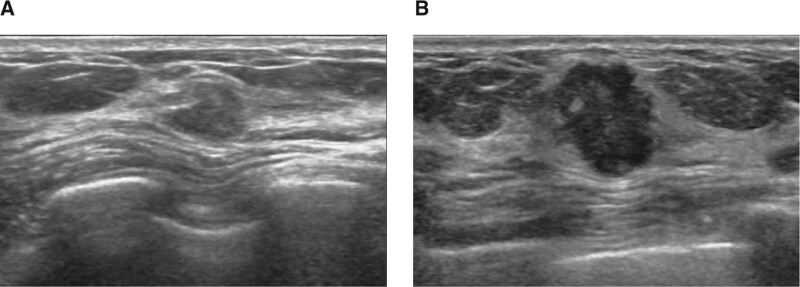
Diagnostic US for mammographic abnormality of a 68-yr-old woman. (A) Prior US shows 0.7 cm sized hypoechoic mass with an irregular shape and microlobulated margin. This mass was initially assessed as BI-RADS category 3 but reassessed as BI-RADS category 4 on the second review due to its shape and margin. (B) An 8-mo follow-up US revealed size increment to 1.2 cm, change in orientation from parallel to nonparallel, and newly developed posterior enhancement observed. This mass was confirmed as invasive apocrine carcinoma. BI-RADS = Breast Imaging Reporting and Data System, US = ultrasound.
